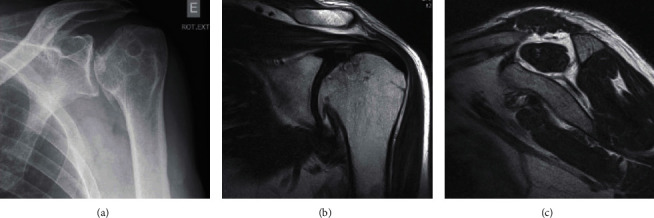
Anteroposterior plain radiograph of the left shoulder with the crescent sign, collapsed humeral head, and osteophyte (a); T2 coronal magnetic resonance image with a collapsed humeral head and large osteophyte (b); T1 magnetic resonance image with grade 1 rotator cuff muscle fatty infiltration (c).
